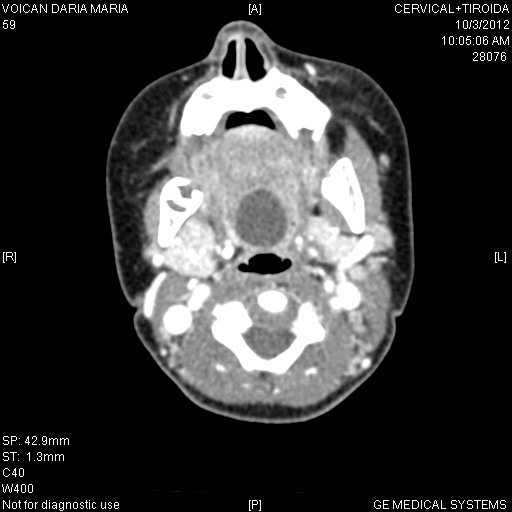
Preoperative CT imaging of case 2
